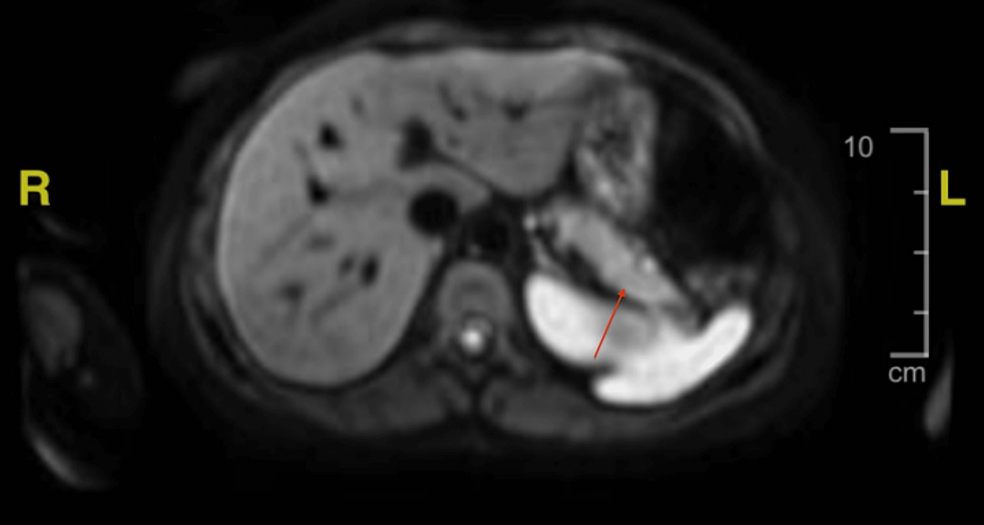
Diffusion restriction within the distal body and pancreatic tail of the pancreas is noted on upper abdominal MRI.
